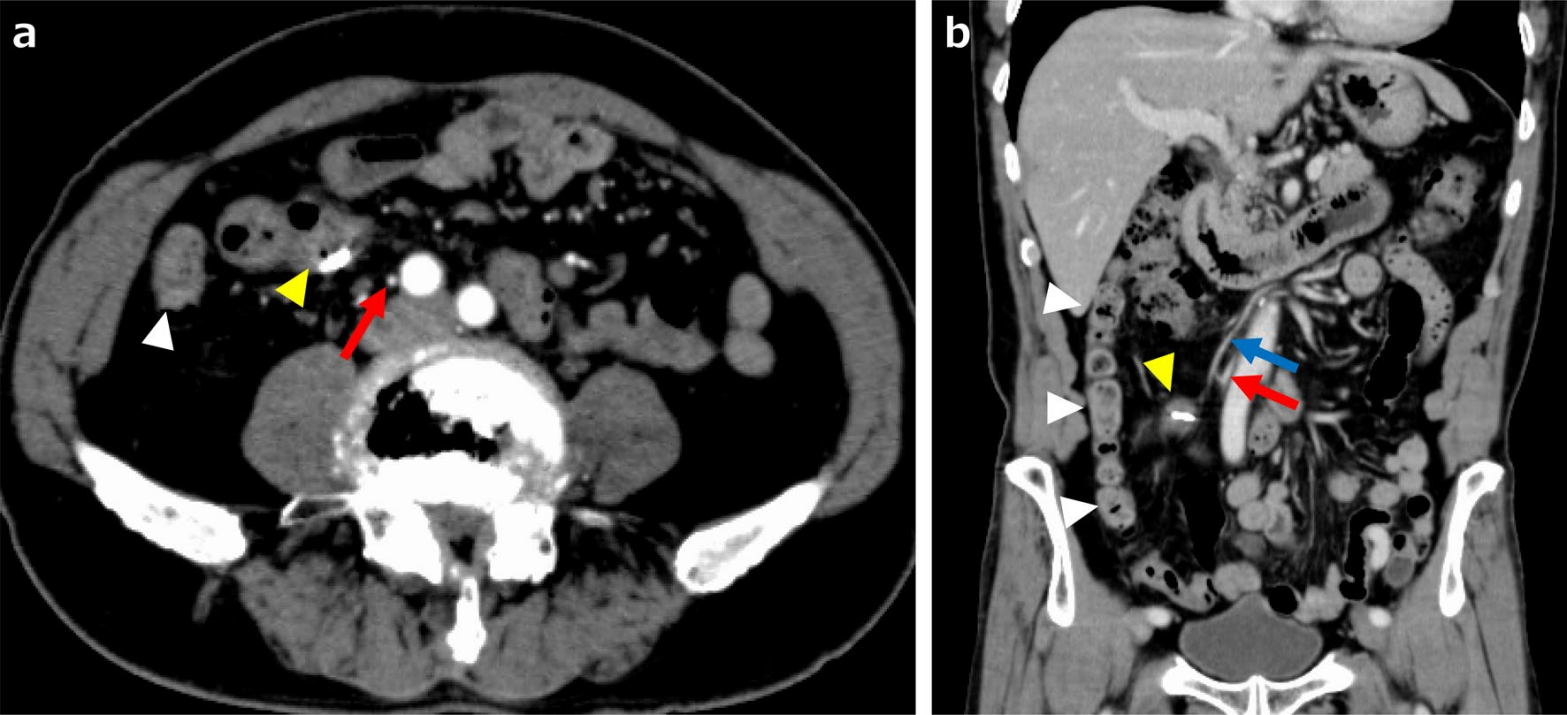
Contrast-enhanced computed tomography (CT) images. a The axial CT image revealed that the clip was located in the descending colon on the right side (yellow arrowhead), the IMA ran symmetrically (red arrow), and the sigmoid colon was located on the right side (white arrowhead). b A sagittal CT image revealing the clip in the right-sided descending colon (yellow arrowhead) and right-sided fixed sigmoid colon (white arrowhead) and symmetrical runs of the IMA (red arrow) and IMV (blue arrow)
